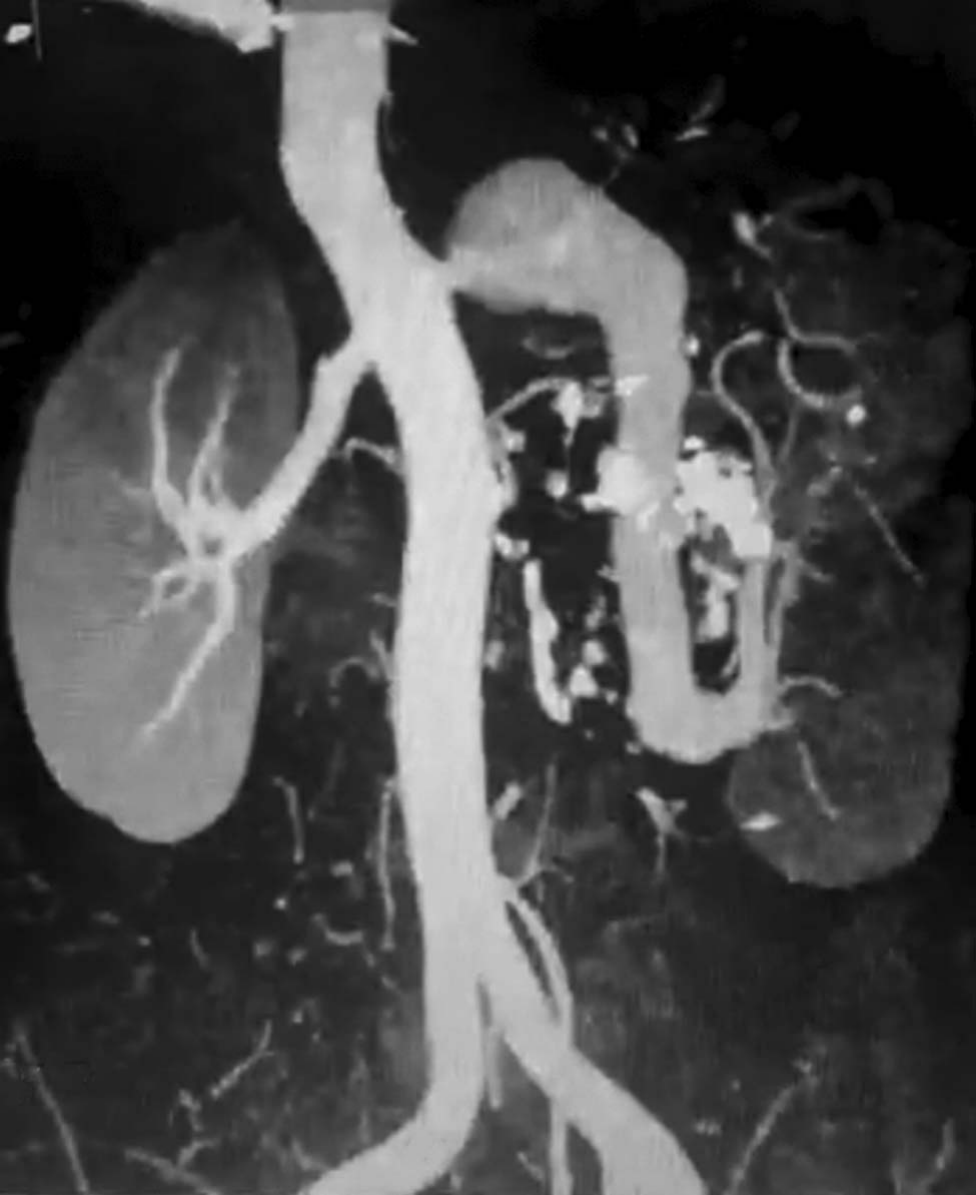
Multi-slice computed tomography angiogram shows a giant and elongated left renal aneurysm. Ectatic dilatation of the upper-lobe renal artery branch is noticed.
